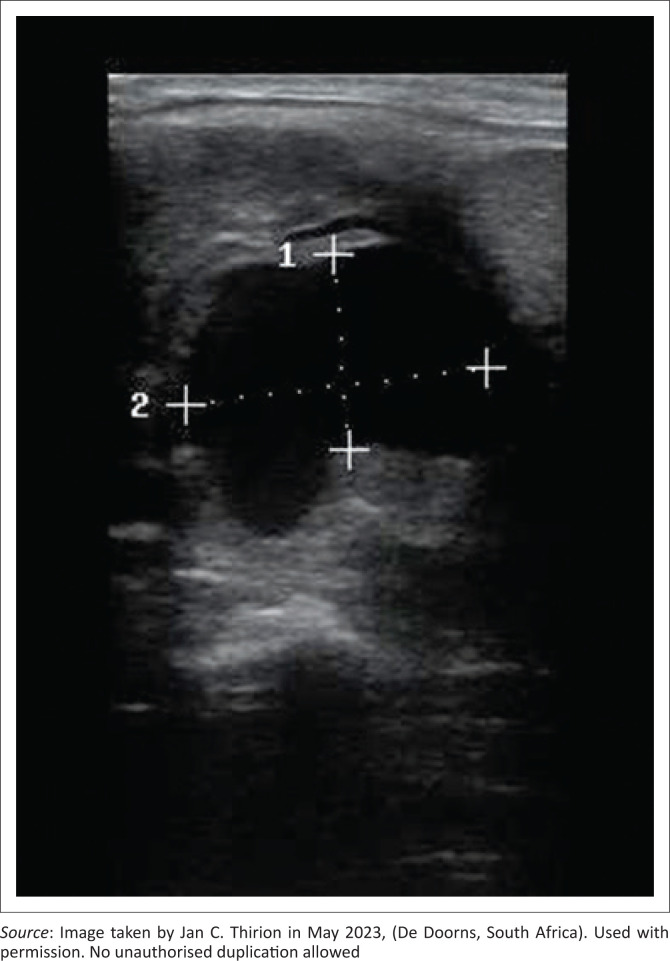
Image of pseudo-aneurysm taken during point-of-care ultrasound examination.
